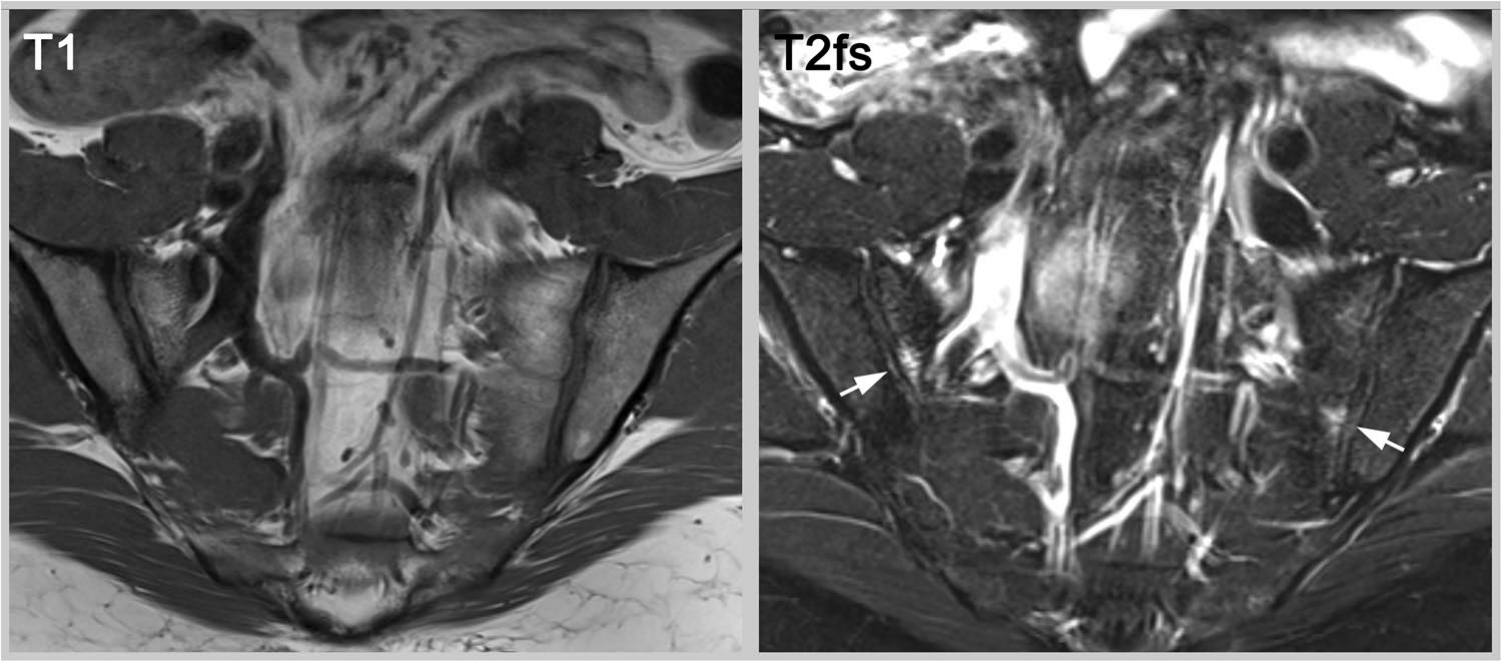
Bone marrow edema in two locations in an asymptomatic female volunteer. The T2w fat saturated (T2fs) sequence shows small bilateral bone marrow lesions (arrows) in an asymptomatic female. Whereas the MRI meets the outdated, quantitative definition of sufficient bone marrow edema for an ASAS-positive MRI, diagnosis is not supported by any structural lesions, and the individual would not fulfill the entry criteria of the ASAS classification system
